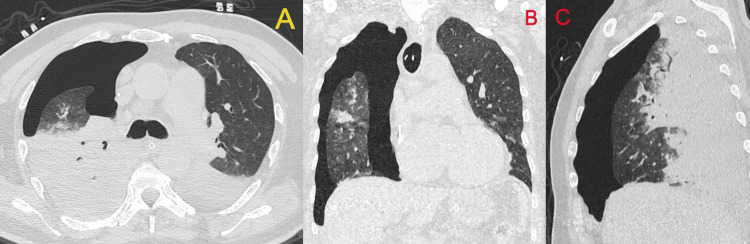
High-resolution computed tomography (HRCT) of the thorax (lung window): large right pneumothorax with compressive atelectasis A (Axial): A large right pleural air collection occupies the anterolateral hemithorax with marked passive collapse of the right lung and dependent basal consolidation with air bronchograms.
